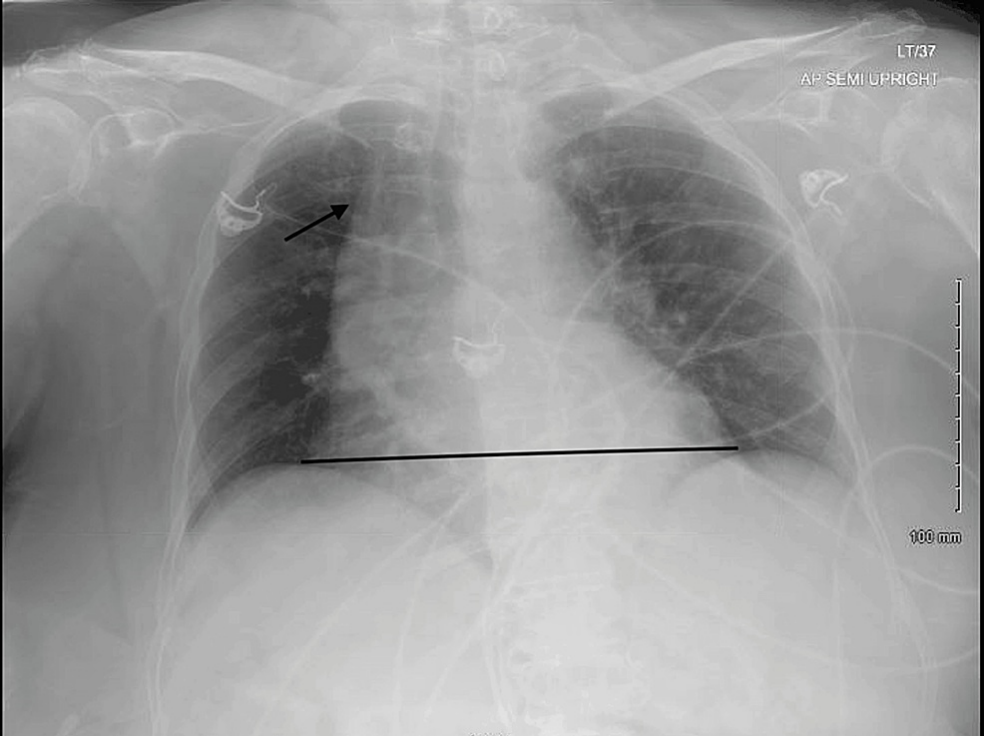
Chest x-ray showing cardiomegaly
